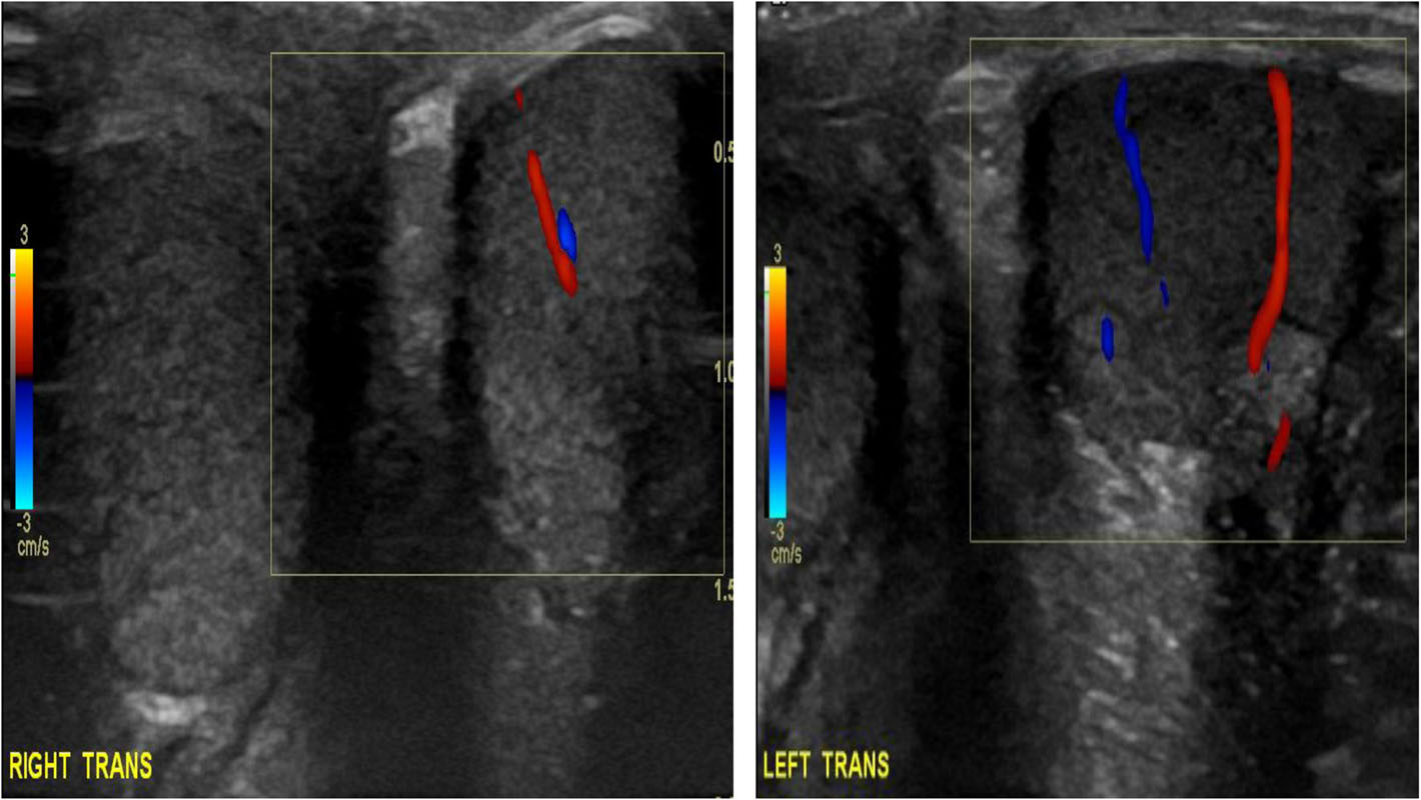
Doppler study of both testes (transverse views) showing globally reduced (but not absent) perfusion in the right testes compared to the left
